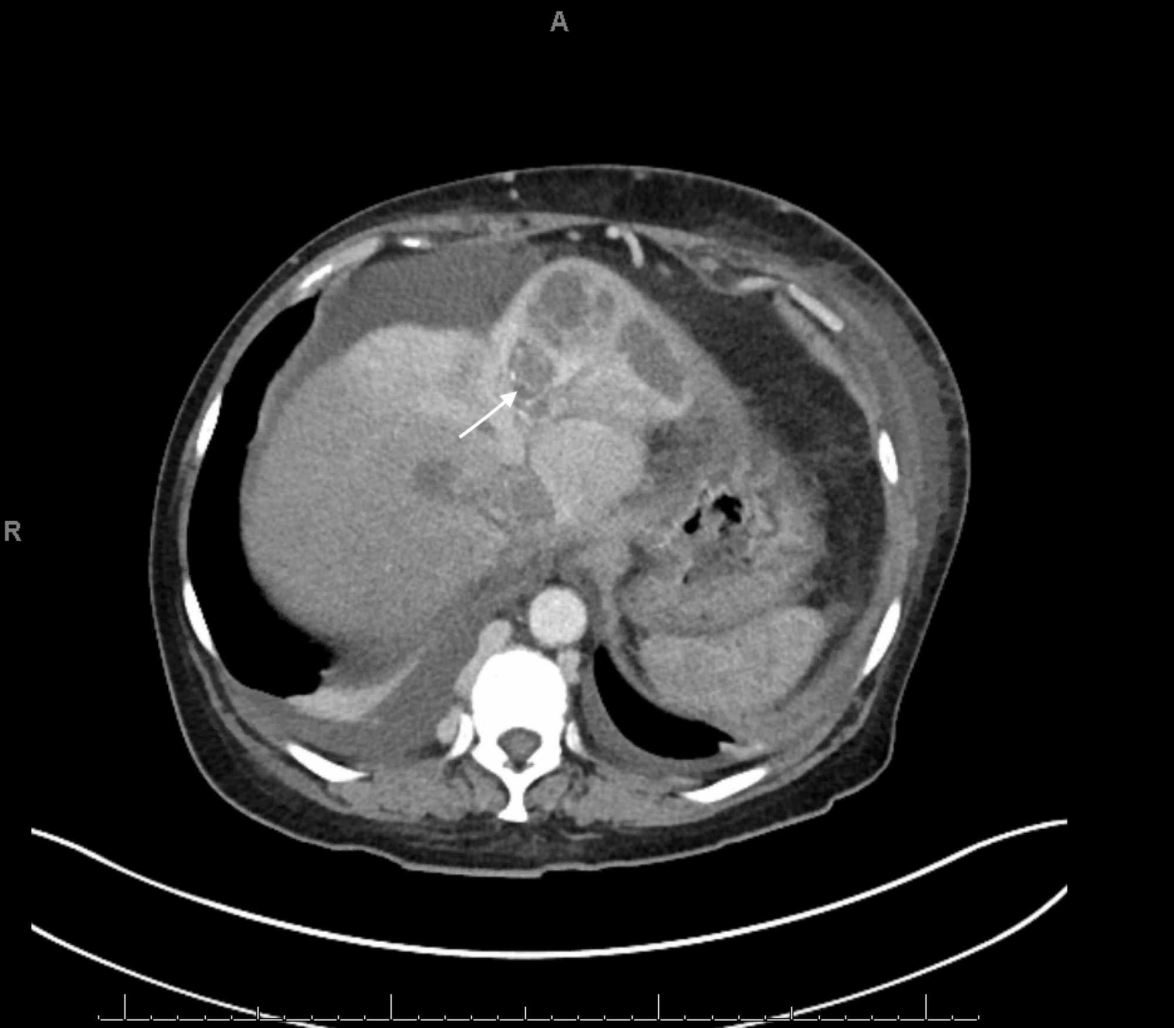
Triple-Phase Liver CT - Transverse section of the Liver Triple-phase liver CT revealed a mass occupying the left hepatic lobe with necrosis of the liver.
